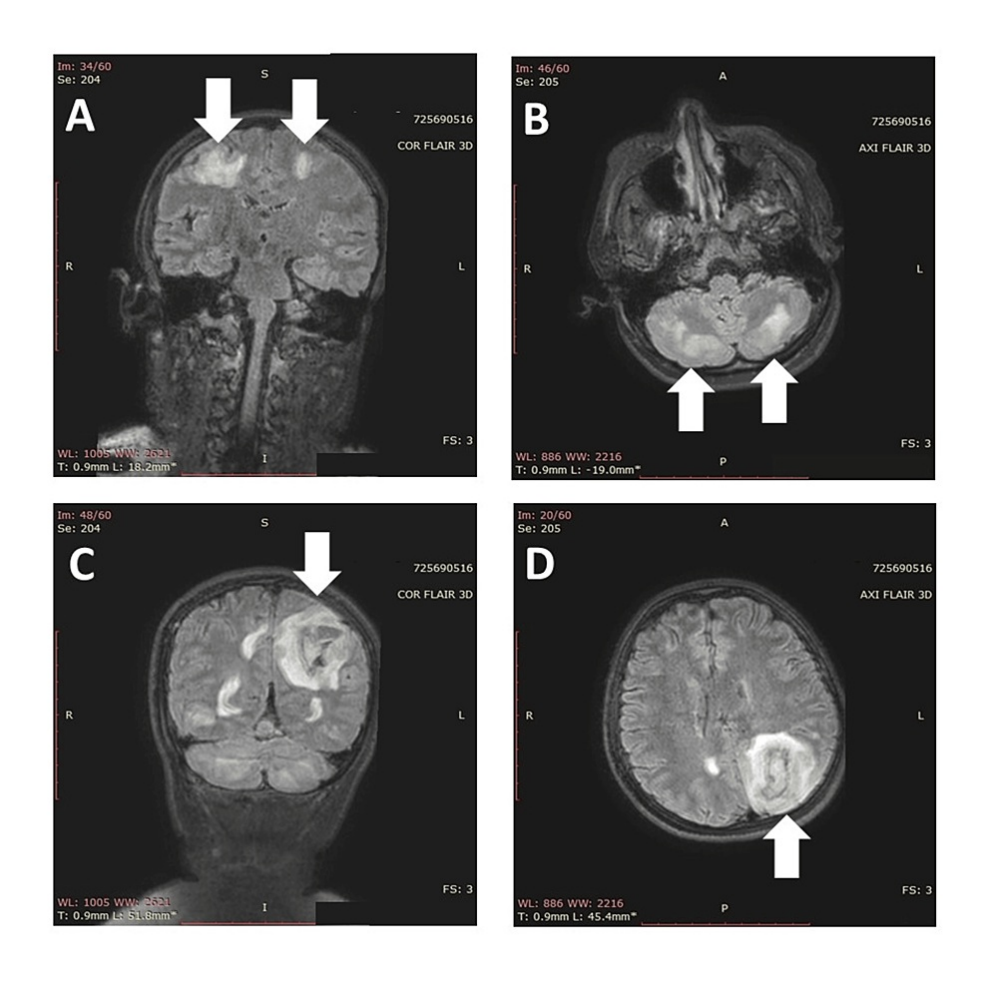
Multiple white matter hyperintense areas on FLAIR without diffusion restriction in the bilateral frontoparietal region (arrows in panel A) and both cerebellar hemispheres (arrows in panel B). Left parieto-occipital intraparenchymal hemorrhage (arrows in panels C and D) FLAIR: fluid-attenuated inversion recovery
